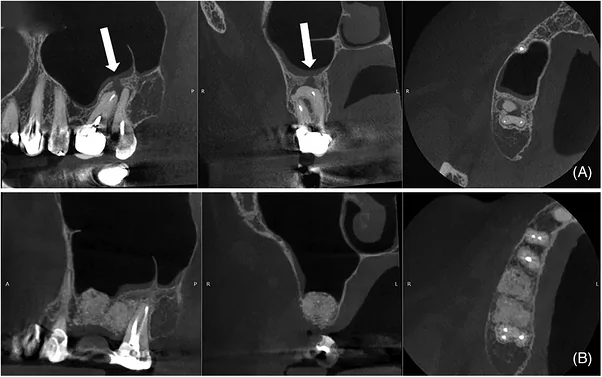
Case 3: Initial CBCT (sagittal, coronal, axial), showing hypodense lesion involving teeth #1 and #2, sinus membrane thickening, cortical fenestration and oroantral communication (arrows) (A). CBCT at 06 months postoperatively (sagittal, coronal, axial), showing bone formation, normalized sinus membrane, cortical repair and closure of oroantral communication (B). CBCT, cone‐beam computed tomography.
